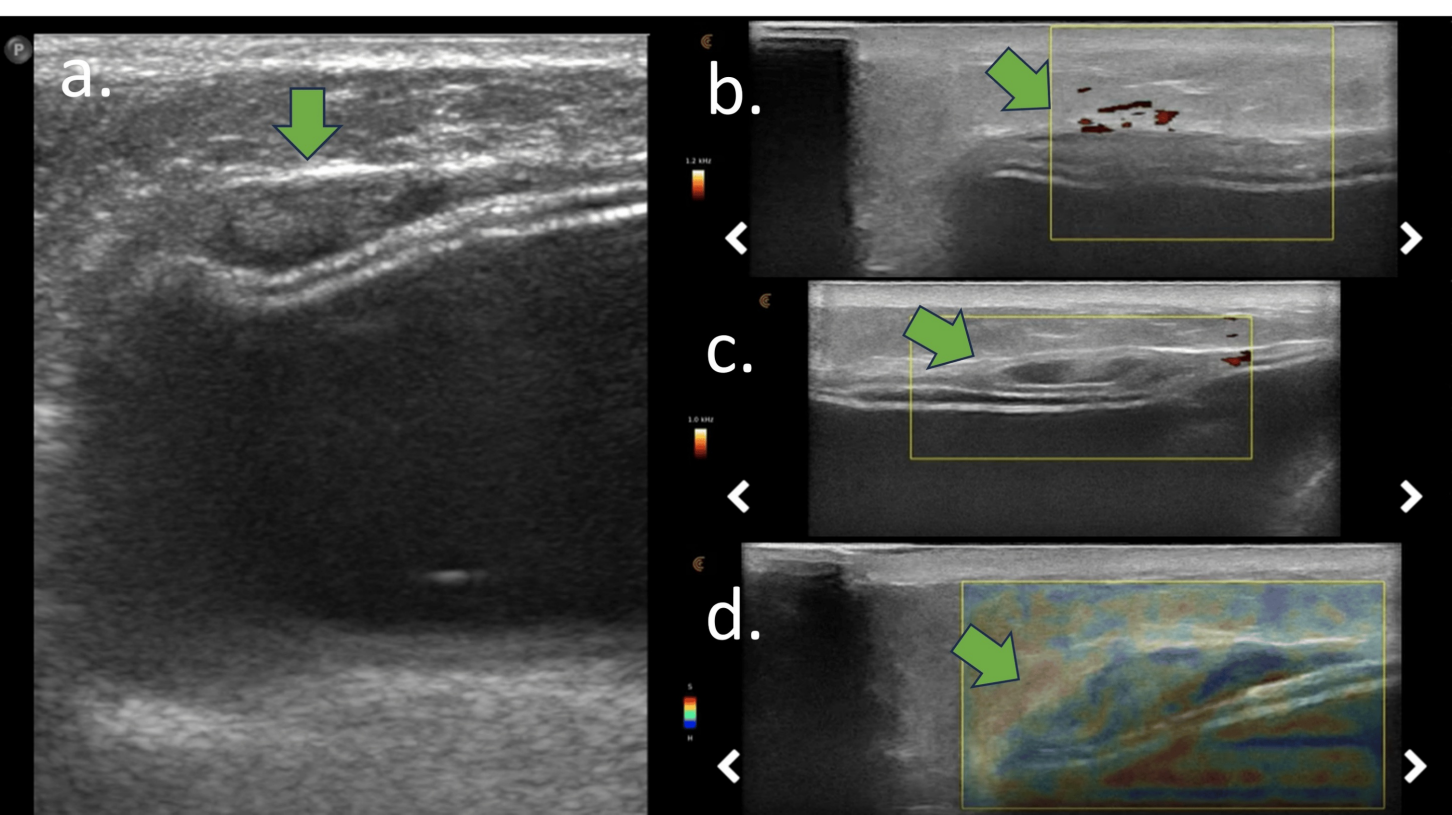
Ultrasound findings of early acellular dermal matrix (ADM) complications USG of the same patient as in Figure 5. (a) B-mode, (b) power-Doppler mode, (c) power-Doppler mode, and (d) elastography. (a, b, c, and d) Intracapsular heterogeneous tissue (green arrow) associated with intracapsular collection and fibrous capsule thickening. (b and c) Increased vascularization in the fibrous capsule. (d) The intracapsular tissue has moderate to high stiffness in elastography.
